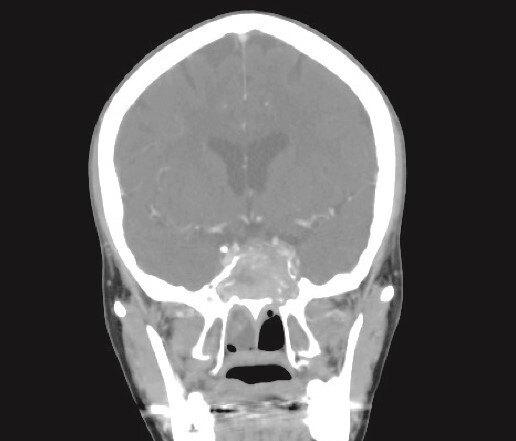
CT brain, coronal reconstruction shows bilateral near total opacification and expansion of the paranasal sinuses and nasal cavity. Invasion of the left cavernous sinus with encasement of cavernous segment of left ICA. Pituitary gland and optic chiasm displaced superiorly.
